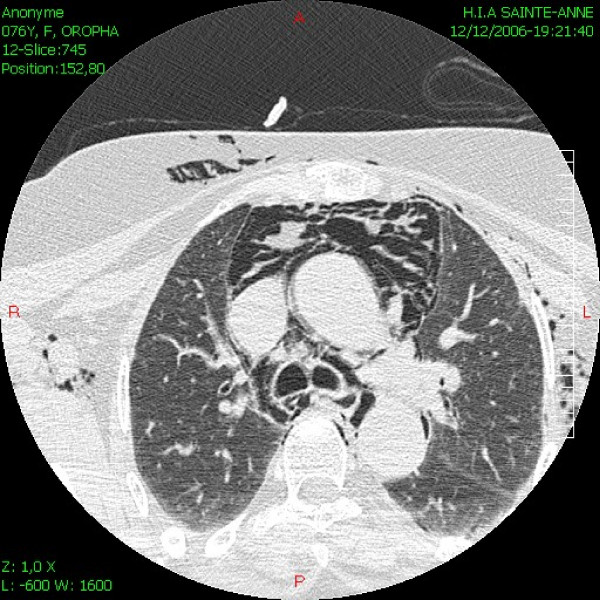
Thoracic CT showing mediastinal emphysema.
